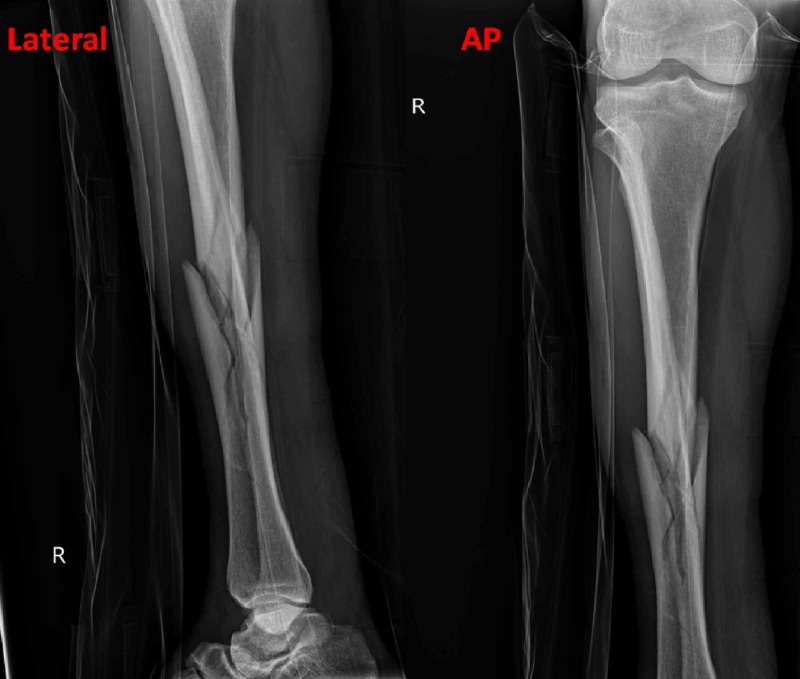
Lateral and anteroposterior (AP) x-ray views of tibia and fibula fracture on presentation
